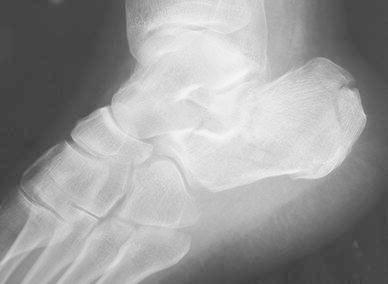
Lateral preoperative radiographs of a Sanders type III thalamic fracture
